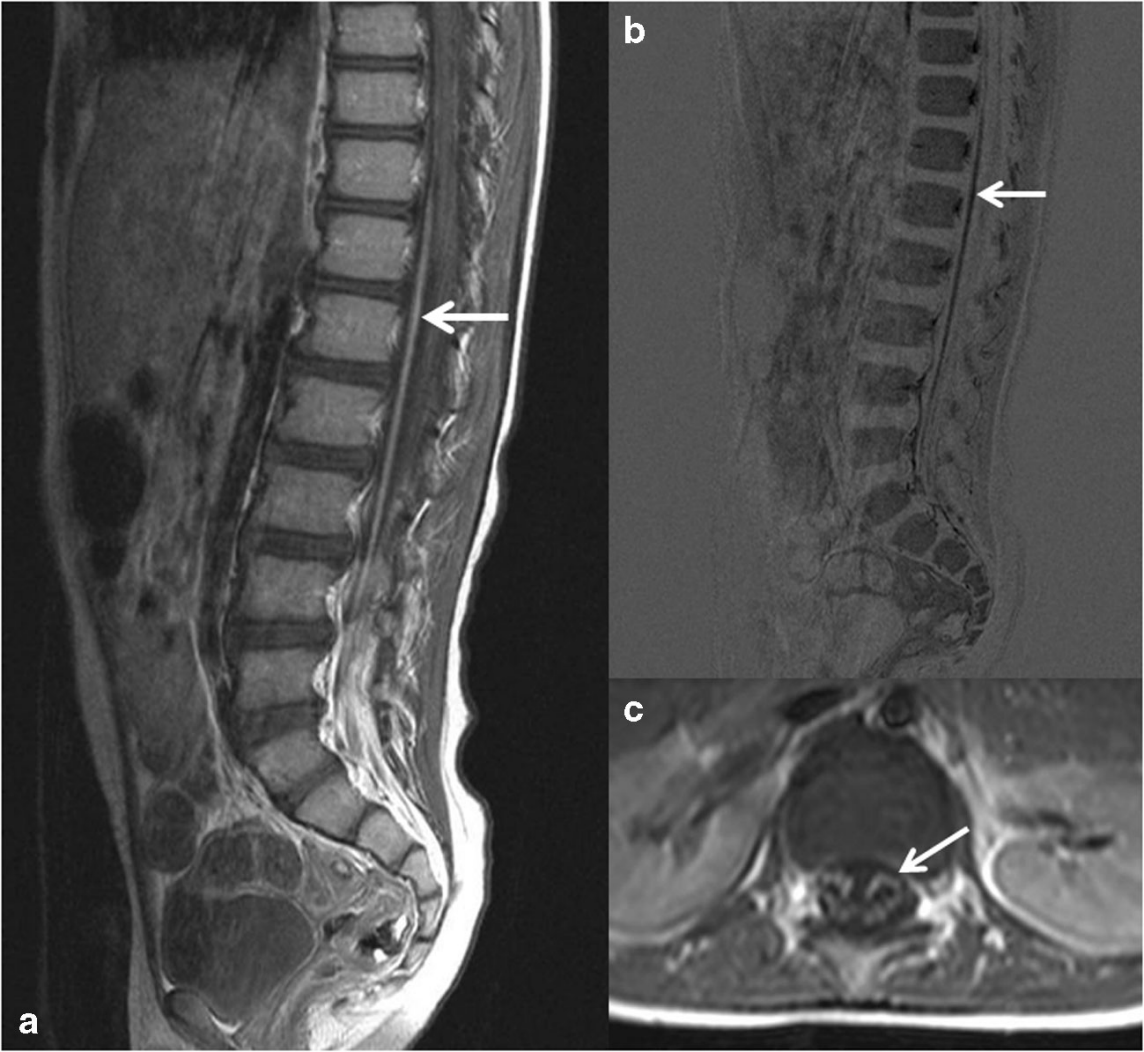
MRI of the spinal cord in a 3-year-old boy with Guillain-Barré syndrome. a Sagittal contrast-enhanced T1 showing typical enhancing anterior caudal roots. b Subtraction of A with more clear depiction of enhancing caudal root. c Transverse T1 showing more clear enhancement of anterior motor roots
